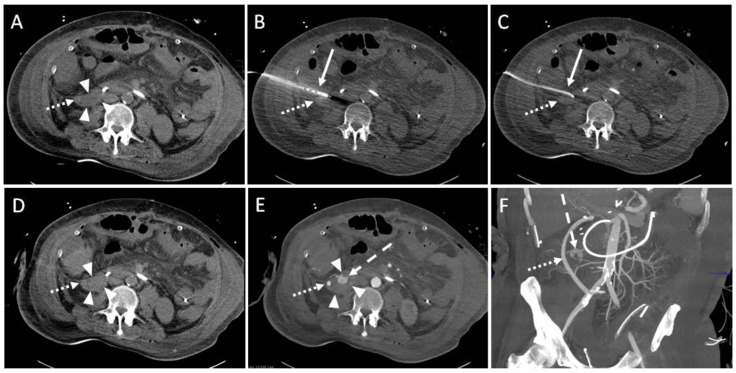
Example of a CT-guided drainage placement with significant complications following SIR guidelines. A 47-year-old male with history of liver transplantation due to alcoholic cirrhosis. After six weeks, an increase in inflammatory parameters was observed. (A) CT planning scan 52 days after transplantation: a fluid collection (arrowheads; density: 6 Hounsfield units (HU)) encompassing the bypass (dotted arrow) between right common iliac artery and hepatic artery in the right paracolic gutter. (B) Using lateral access via the retrocolic space, a 10F pigtail drainage (solid arrow) is placed under CT fluoroscopy. Dotted arrow: drainage trajectory anterior to the bypass. (C) After removal of the Trocar, a correct position of the drain (solid arrow) was shown. Several milliliters of serous fluid could be aspirated. Dotted arrow: bypass. (D) An unenhanced CT control scan revealed increased density (30 HU; compare to figure (A)) of the fluid collection (arrowheads) in the right paracolic gutter suspicious of acute bleeding. Dotted arrow: bypass. (E) Enhanced follow up CT scan 10 min after drain placement shows contrast agent extravasation (dashed arrow) originating from a branch of the right colic artery. Due to the arterial contrasting, the bypass (dotted arrow) is now clearly delineated. Arrowheads: fluid collection. (F) Coronary maximum-intensity projection (40 mm) showed an intact, inconspicuous bypass (dotted arrow). Dashed arrow: contrast agent extravasation. The injured vessel was subject to successful immediate revision surgery.
